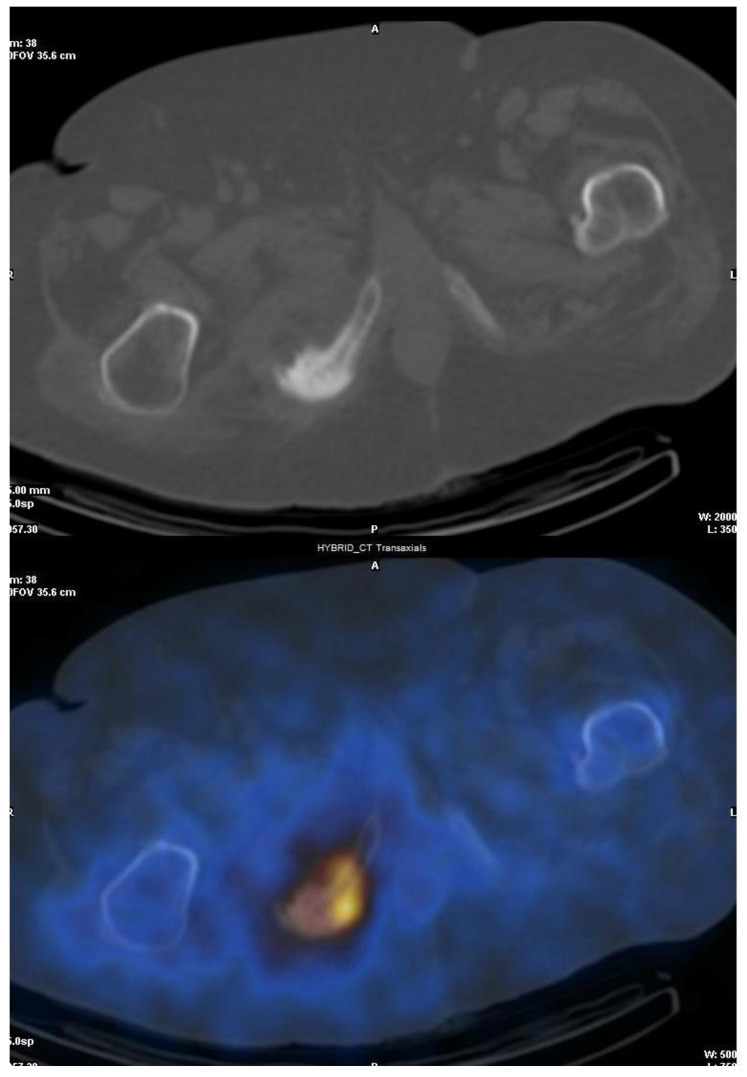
Osteomyelitis of the ischiopubic ramus by *Morganella morganii* and *Proteus mirabilis*. A 73-year-old male patient with a previous diagnosis of a polymicrobial stage 4 bedsore over ischial tuberosity who developed an osteomyelitis of the ischiopubic ramus and secondary bacteremia by *Morganella morganii* and *Proteus mirabilis*. A SPECT-CT with ^67^Ga was performed showing pathological gallium deposits on the right ischiopubic ramus.
